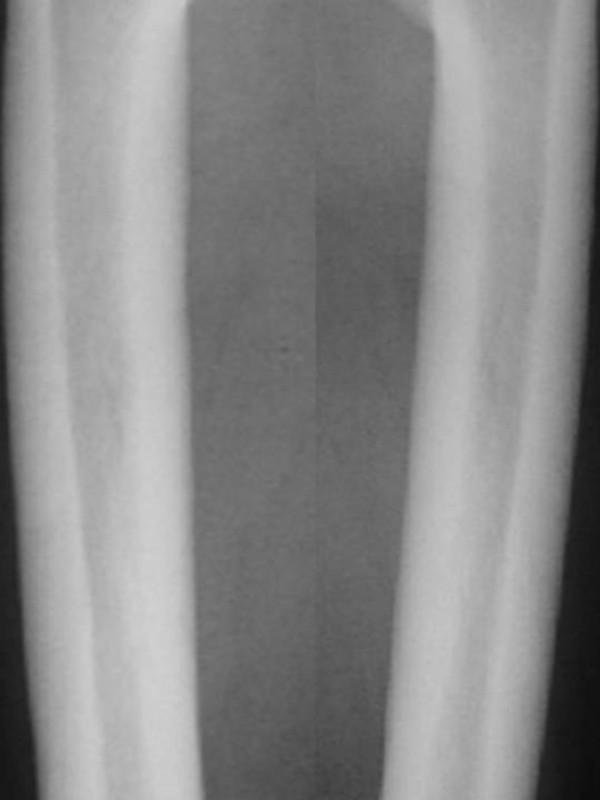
(patient 2). Radiographic examination of the femora showed thick, and dense, endosteal hyperostosis more marked at the mid-diaphyses. The medullary canals were narrow but patent, and the endosteal surface was irregular (arrow).
